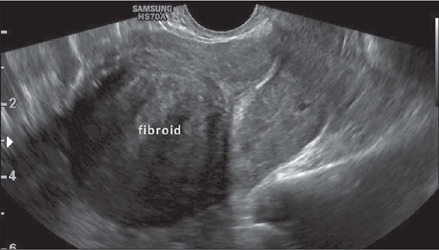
Transvaginal ultrasound image, in a longitudinal view, showing a hybrid (FIGO 2-5)
